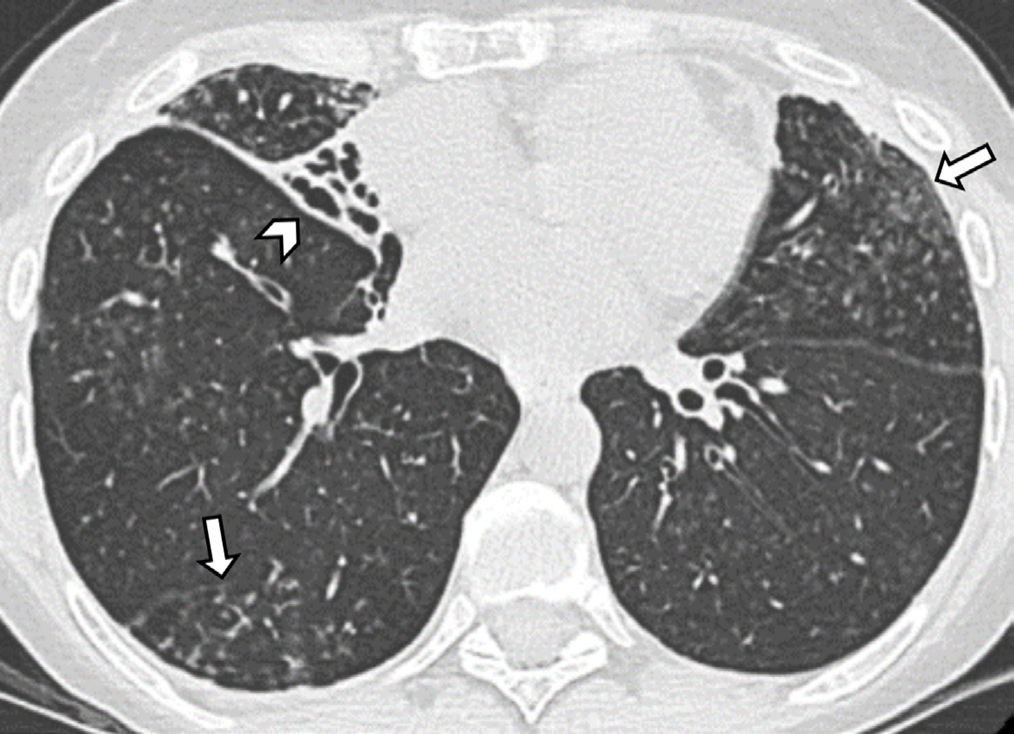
Axial CT image in a patient diagnosed with non-tuberculous mycobacterial infection shows varicoid bronchiectasis and volume loss in the middle lobe (arrowhead). Bilateral centrilobular nodules and tree-in-bud opacities (arrows) due to distal airways involvement can be appreciated in the right lower lobe and in the left upper lobe.
